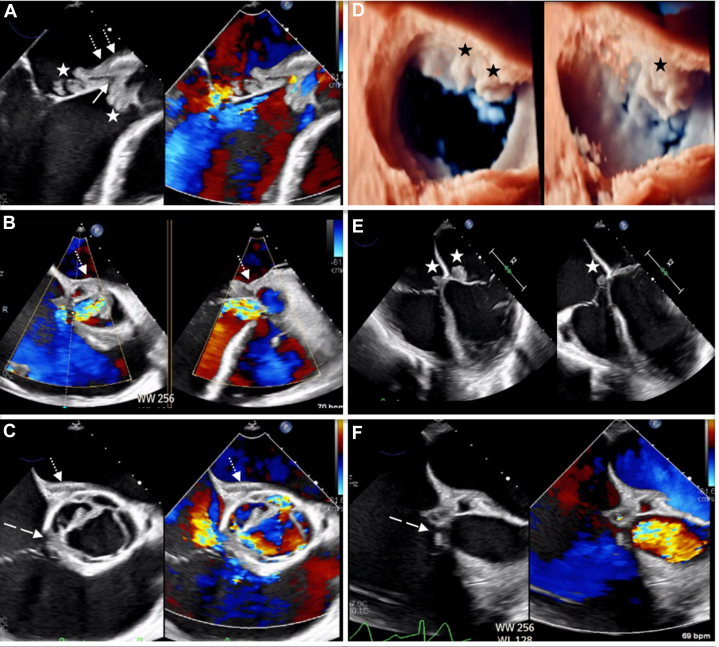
Preoperative Transesophageal Echocardiography (A, B, C, and F) Images show a large aortic root abscess (dotted arrows) causing noncoronary cusp perforation, right atrial fistula (dashed arrows), and severe aortic regurgitation. Also, multiple vegetations (stars) of the noncoronary aortic leaflet, anterior mitral valve leaflet, and septal tricuspid valve leaflet (A, D, and E) are seen, while perforation of the base of the anterior mitral leaflet is suspected (solid arrow, panel A).
